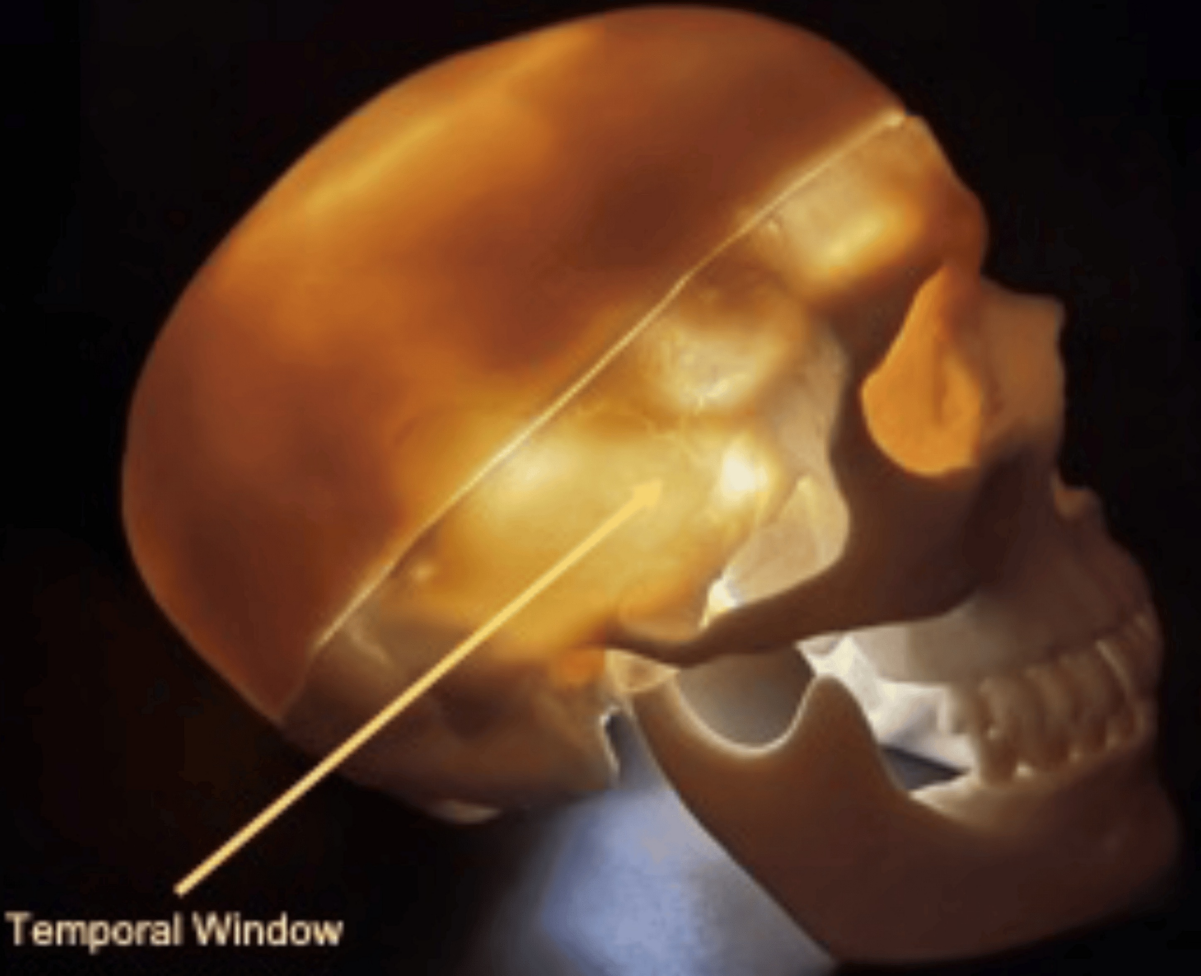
A plastic skull model used to demonstrate the relatively thin temporal bone window used for BrainPOCUS. BrainPOCUS: Brain point-of-care ultrasound
